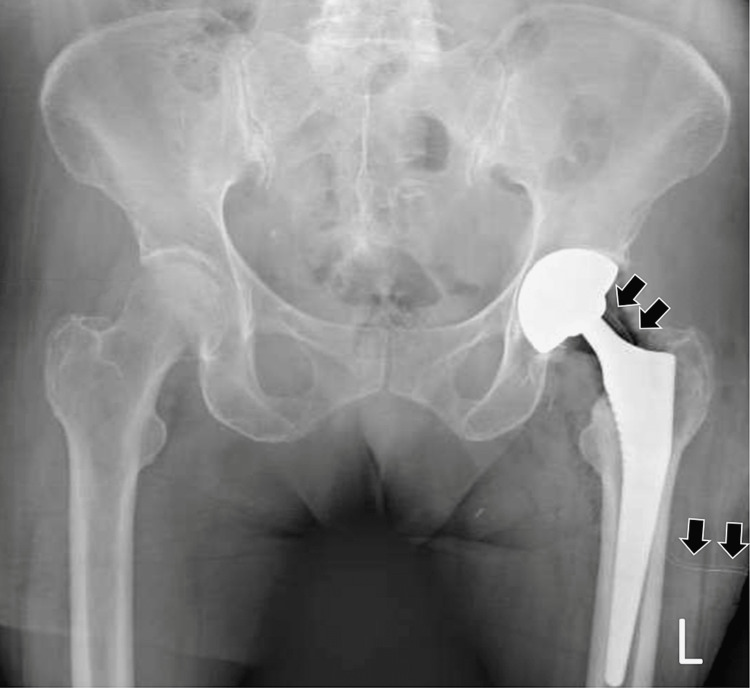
Immediate postoperative radiograph following total hip arthroplasty. Standard anteroposterior hip and pelvis radiograph taken immediately postoperatively. Notice the position of the vacuum drainage tube (as depicted by black arrows) and its course toward the posterior-inferior aspect of the dual-mobility cup.
